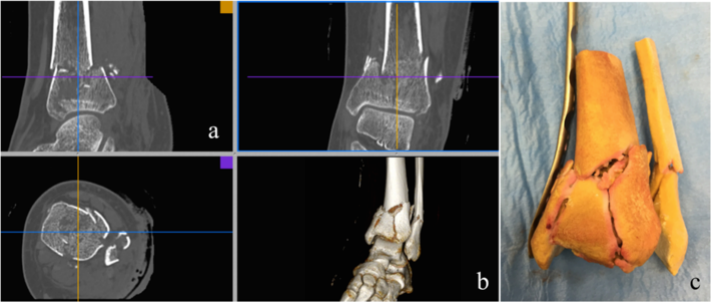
2D CT scan (a), 3D Volume rendering (b) 3D Printed Replica of an ankle fracture with sterile model on the surgical table for plate testing (c)
